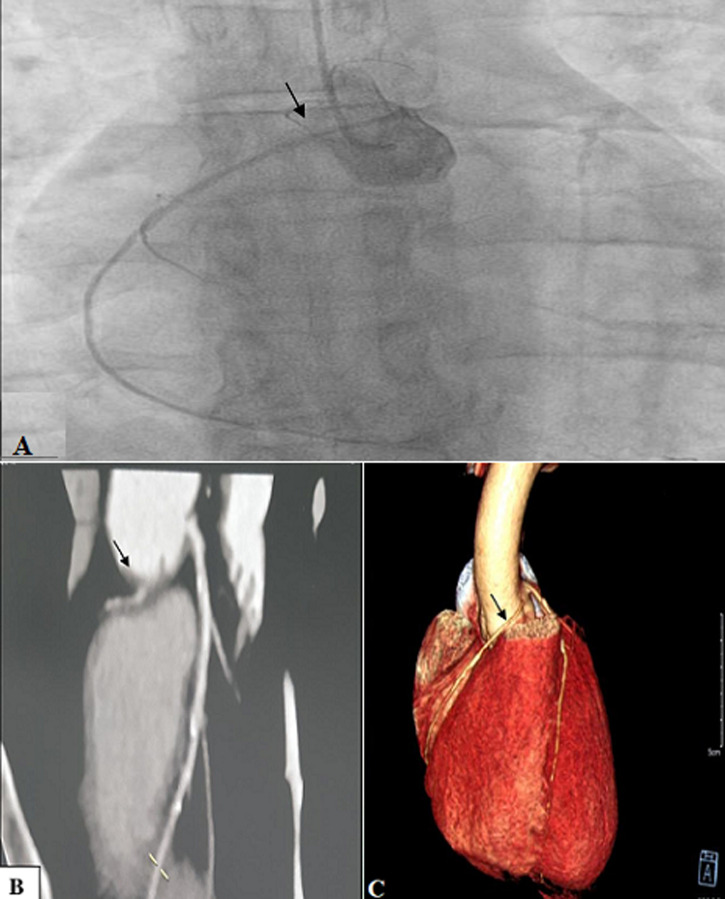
patient #2; (A) angiographic image depicting an ectopic connection of the right coronary artery to the left sinus (arrow); (B, C) CT images showing an unusual connexion of the right coronary artery 7.3mm in front of the birth of the common trunk (arrow) with a proximal inter aorto-pulmonary path
